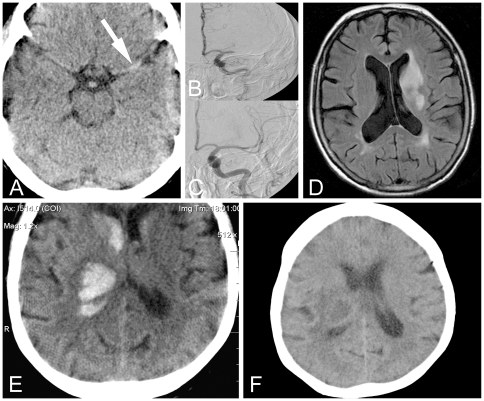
Brain imaging in prehospitally treated stroke patients . Patient with ischemic stroke: Prehospital CT excluded contraindications for thrombolysis as a precondition for prehospital rt-PA thrombolysis (A). The “hyperdense middle cerebral artery” sign (arrow) suggested middle cerebral artery occlusion that was later confirmed in hospital by angiography (B), and reopened by intraarterial recanaliziation (C). Diffusion-weighted magnetic resonance imaging at day 7 showed the residual infarction (D). Patient with hemorrhagic stroke: Prehospital CT scan allowed immediate diagnosis of intracerebral hemorrhage with ventricular extension (E), as a precondition for prehospital differential blood pressure management and telemedicine consultation with hospital experts. CT performed at day 24 shows the residual lesion (F).
